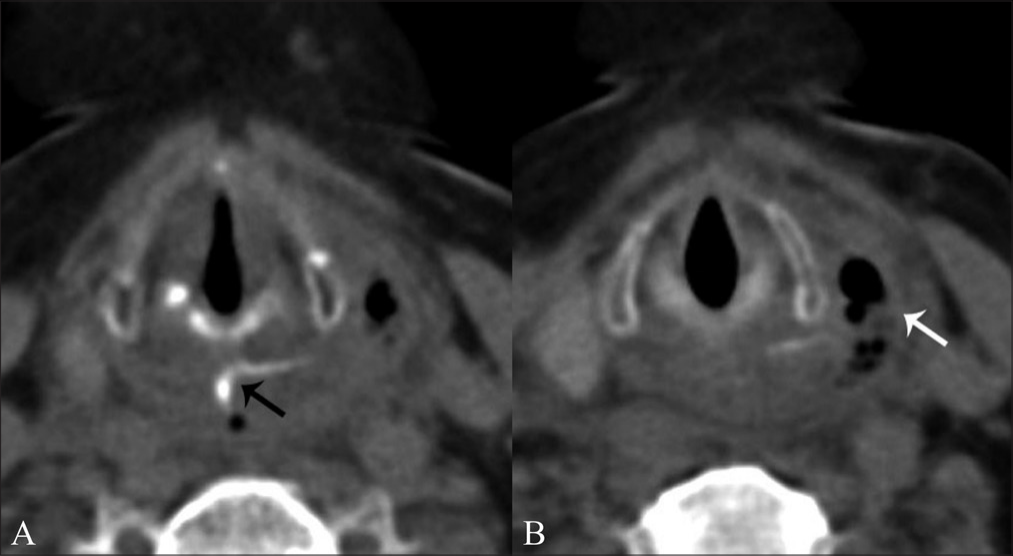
Noncontrast axial CT scans show a curvilinear FB (black arrow in A) at the level of the cricopharyngeus. There is perforation of the pharyngeal wall on the left side with a small parapharyngeal abscess (white arrow in B)
